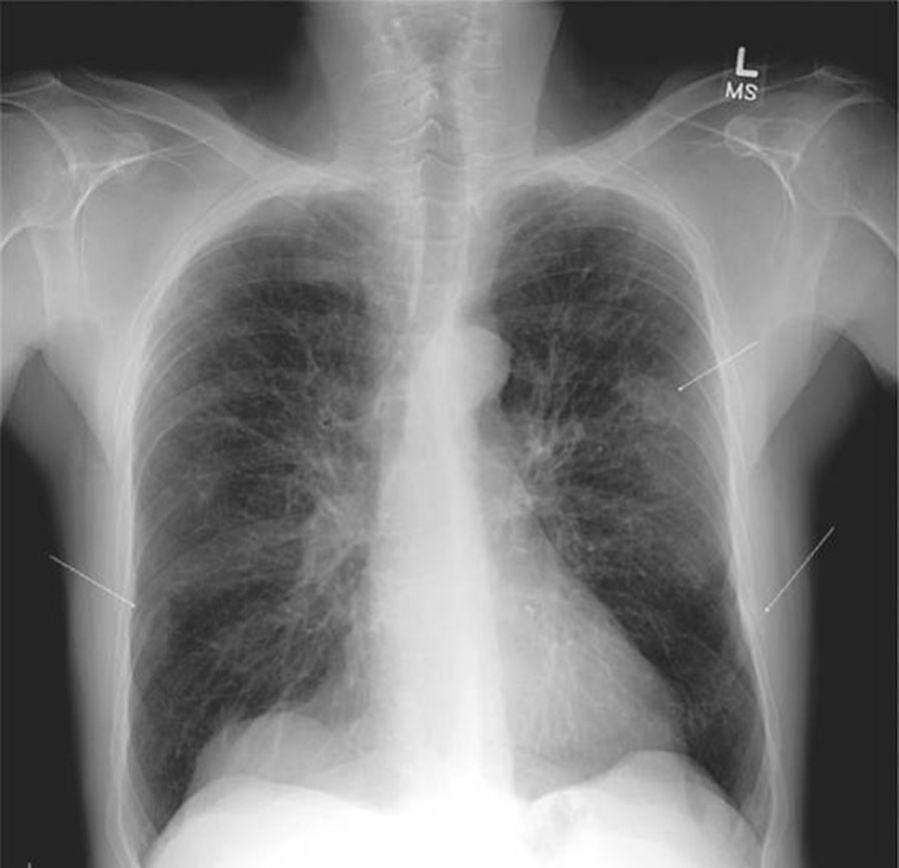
Chest X-ray showing numerous rib fractures
